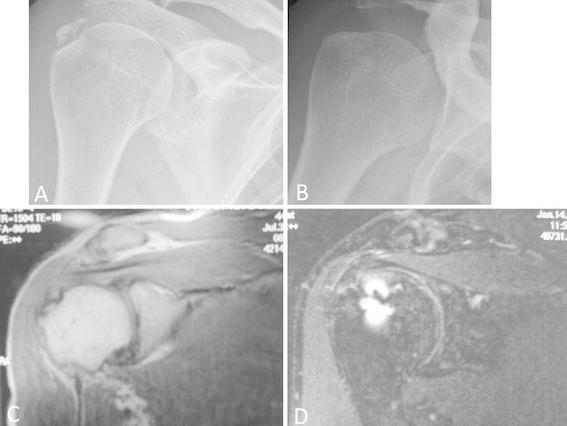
Radiographic evaluation of case 2: a preoperative X-ray (April 2006) of the right shoulder with a dense S/A calcification sized 1.5 cm; b postoperative radiographs showed the complete excision of the calcific deposit; c preoperative MRI (September 2007) before the second operation showed an area of high intensity (T1-weighted) at the supraspinatus insertion that was interpreted as recurrence of calcifying tendinitis with partial tendon tear; d MRI before the third operation showed high intensity in the area where the supraspinatus was fixed, but the tendon appeared healed
